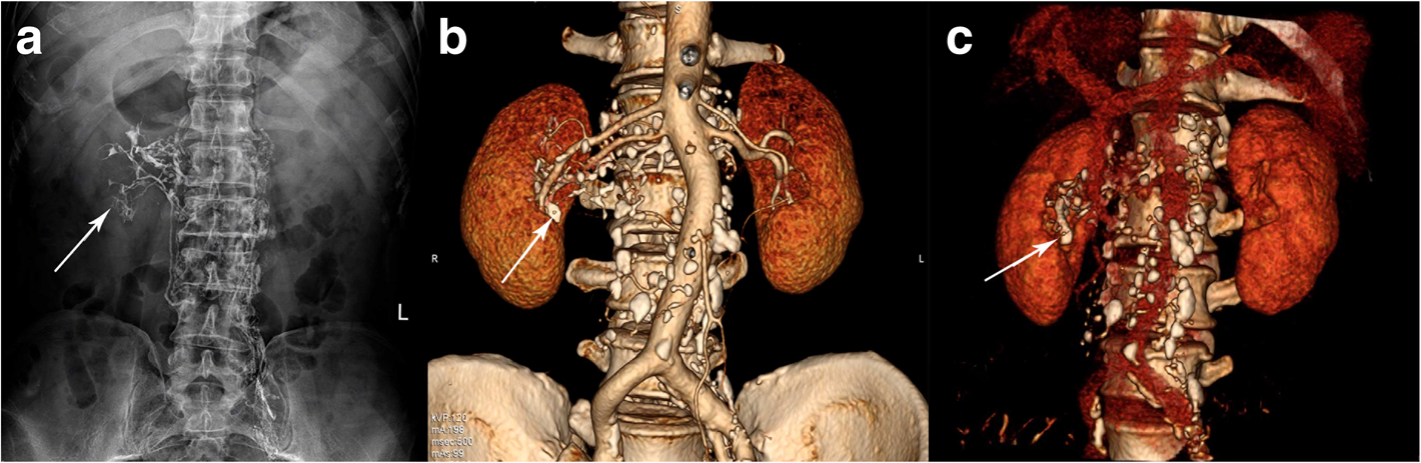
A 60-year-old man with persistent idiopathic chyluria. a Pre-CTA LPG showing wire-like, semicircular shadow at the renal pelvis area and parenchyma in LPG (KUB) indicated right renal lymphatic leakage. b, c LPG CTA showing accumulation of contrast agent in right renal hilus
